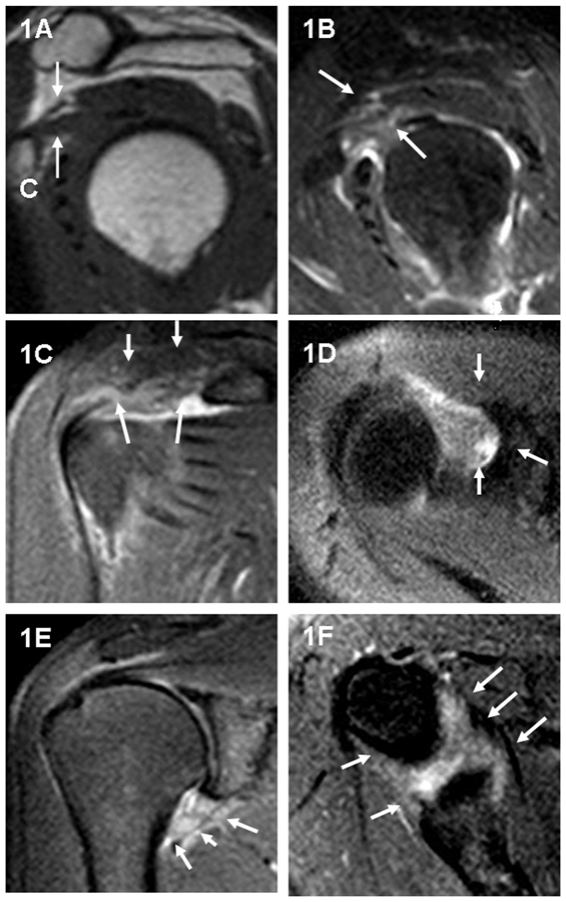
A 56-year-old female patient with clinical evidence of a right frozen shoulder. Sagittal oblique T1-weighted image (TR/TE = 550 ms/15 ms) (1A) shows thickened CHL (arrows). C = inferior margin for the coracoid process. Sagittal oblique (1B), oblique coronal (1C), and transverse (1D) fat-suppressed, proton density weighted, spin-echo image (TR/TE = 3000 ms/34 ms) show high-signal intensity soft tissue in the rotator cuff interval for the same patient (arrows). Coronal oblique (1E) and transverse (1F) fat-suppressed, proton density, weighted spin-echo image (TR/TE = 3000 ms/34 ms) demonstrate a thickened inferior glenohumeral ligament (axillary recess) for the same patient (arrows).
